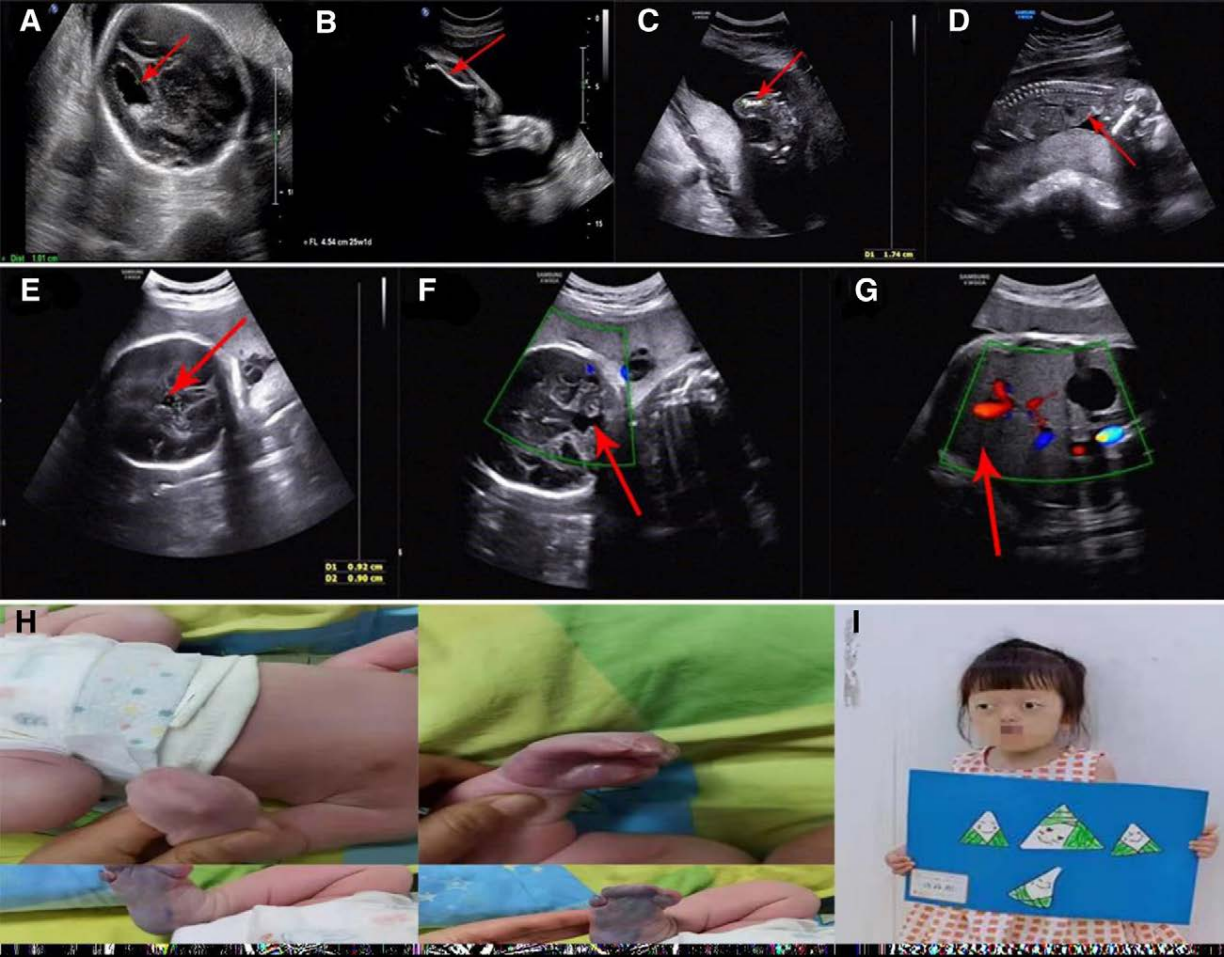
Clinical features of 4 cases. Case 1: (A) the mild dilation of the left ventricle (10.1 mm) in ultrasound; (B) “telephone receiver shape “curved femurs and the length of fetal femur was 4.54 cm at a gestation of 30 weeks. Case 2: (C) ultrasonography indicated short diameter lines of long bones (FL 17.4 mm) at 17w + 4d; (D) “bell-shaped” narrow thorax at 18 w + 4 d. Case 3: (E) intracranial cystic structure about 9.2*9.0 mm; (F) hypoplasia of cerebellar vermis; (G) indistinct gallbladder; (H) tight fusion of all fingers and the II-V toes symmetric syndactyly. Case 4: (I) appearances as Crouzon syndrome with skull and facial deformities, ocular hypertelorism, wide toes. FL = femur length.
